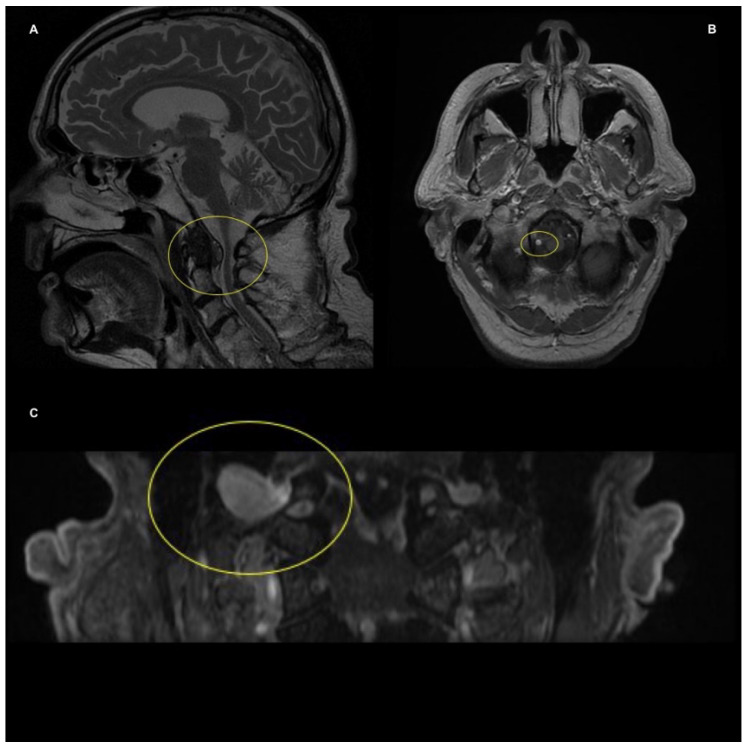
(A). Sagittal T2-weighted MRI image details a hyperintense synovial cyst adjacent to the right side of the craniocervical joint; (B). Axial T1-weighted image shows a hypointense mass in the posterior cranial fossa; (C). Coronal T1-weighted MRI image provides references on the size of the cyst.
